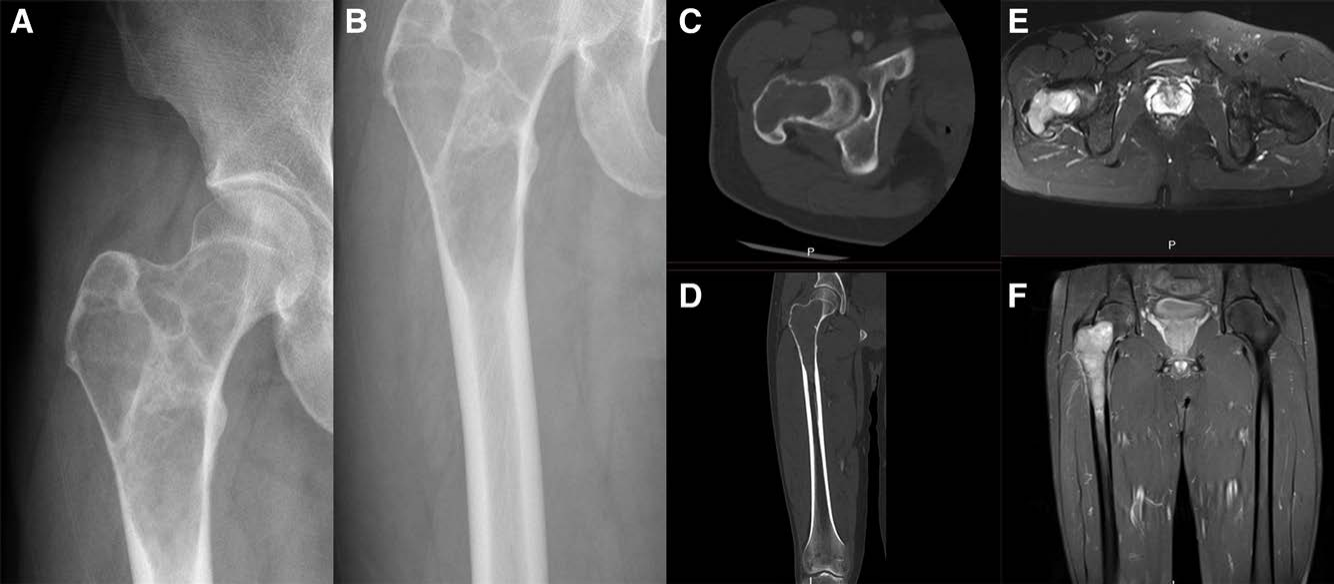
(A and B) Preoperative anteroposterior radiographs showed extensive cystic lesions in the subtrochanter, neck, and intertrochanter of the femur. (C and D) Preoperative computer-enhanced tomography showed no pathological fracture of the proximal femur, and the cortical bone was damaged but intact. (E and F) Preoperative magnetic resonance imaging showed that the lesion did not infiltrate the surrounding soft tissue.
